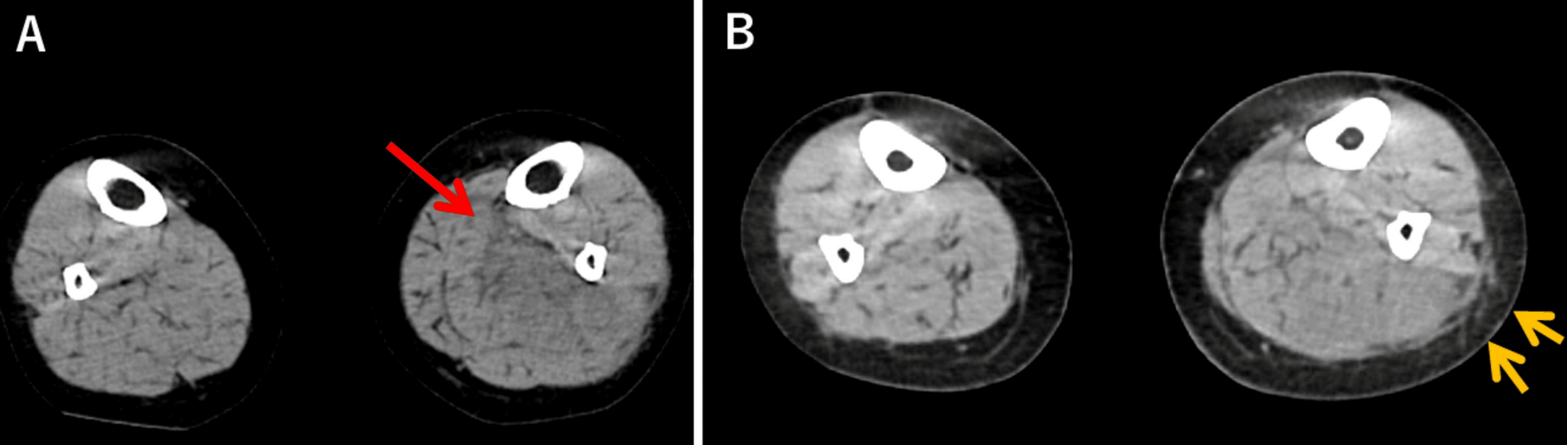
Plain CT of the lower limbs reveals (A) an enlarged left soleus muscle with decreased density (red arrow) and (B) increased density in the perifascial fatty tissue (yellow arrows).
